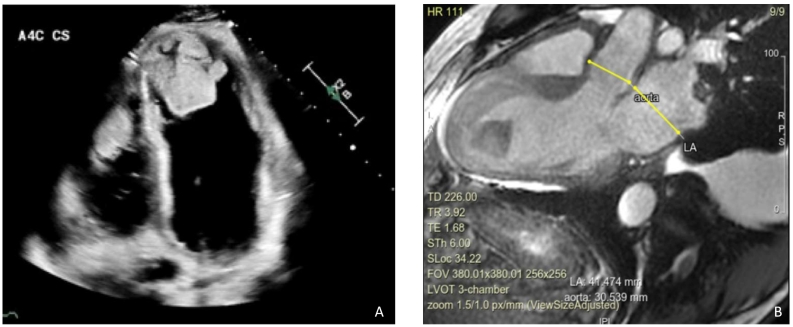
(A) Transthoracic echocardiography showing a severely dilated left ventricle with biventricular thrombi. (B) Cardiac magnetic resonance imaging shows scars in both right ventricle and left ventricle with nonviable myometrium in half of the left ventricle.
